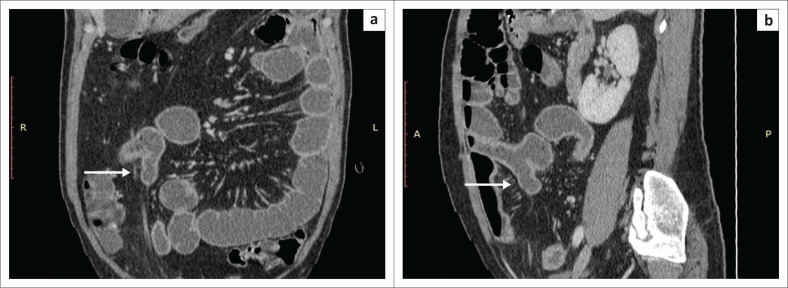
Contrast enhanced computed tomography in the coronal (a) and sagittal (b) planes demonstrating a tubular, blind-ending structure arising from antimesenteric border of the distal ileum with enhancing walls, suggestive of Meckel’s diverticulum (white arrows). No abnormal wall thickening or surrounding fat inflammation was present.
